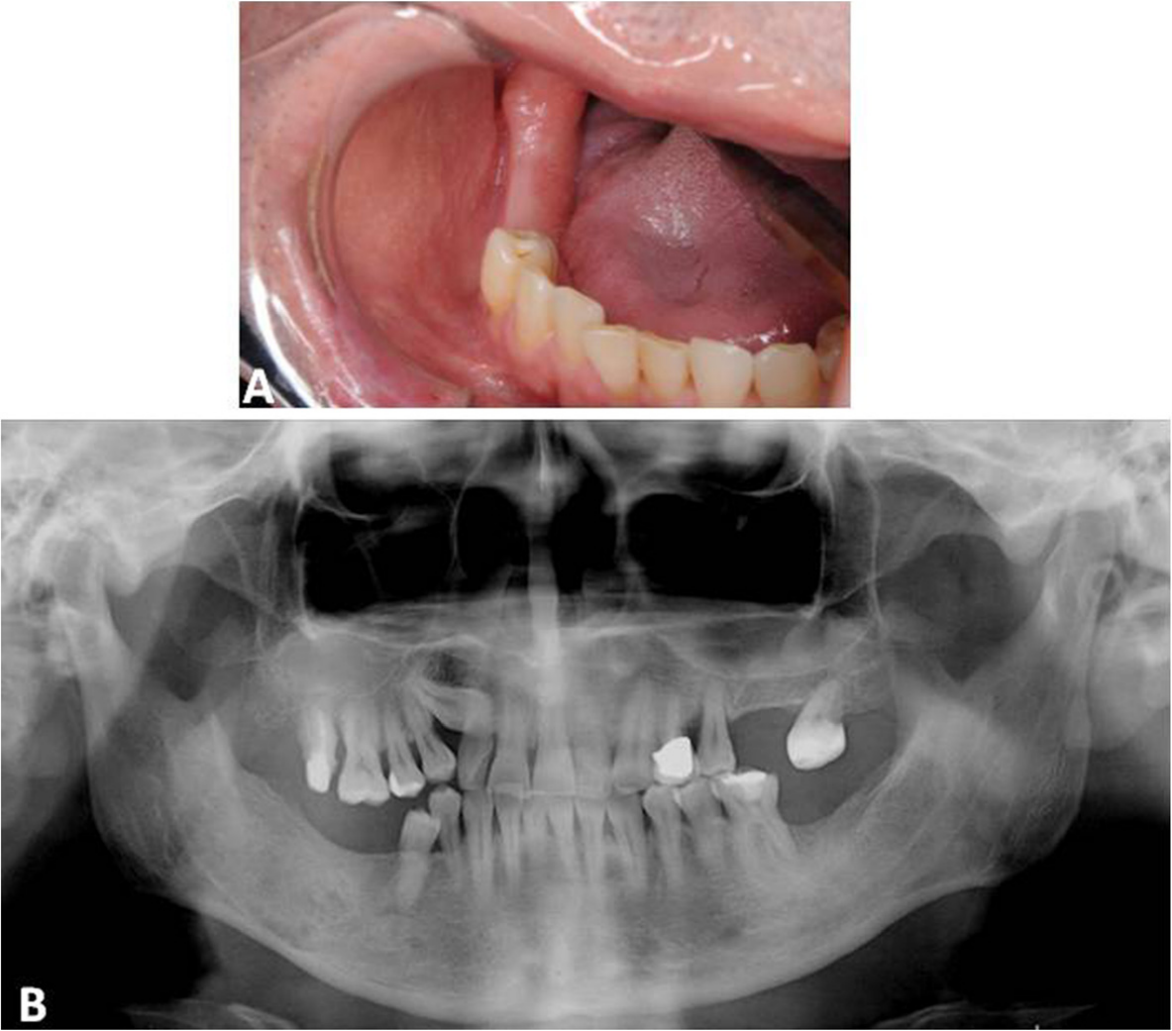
Post-operative findings: Intraoral photograph (A) and panoramic radiograph taken 10 years after surgery indicates full healing without recurrence.
